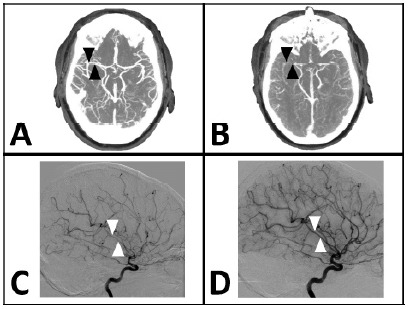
(A) Computed tomography angiography (CTA) of the head upon initial presentation demonstrating the normal-appearing middle cerebral artery (black arrows) compared to the repeat CTA head; (B) demonstrating segmental vasoconstriction (black arrows); (C) cerebral digital subtraction angiography (DSA) demonstrating segmental vasoconstriction (white arrows) compared to DSA immediately following intra-arterial verapamil; and (D) resolution of the vasoconstriction (white arrows).
